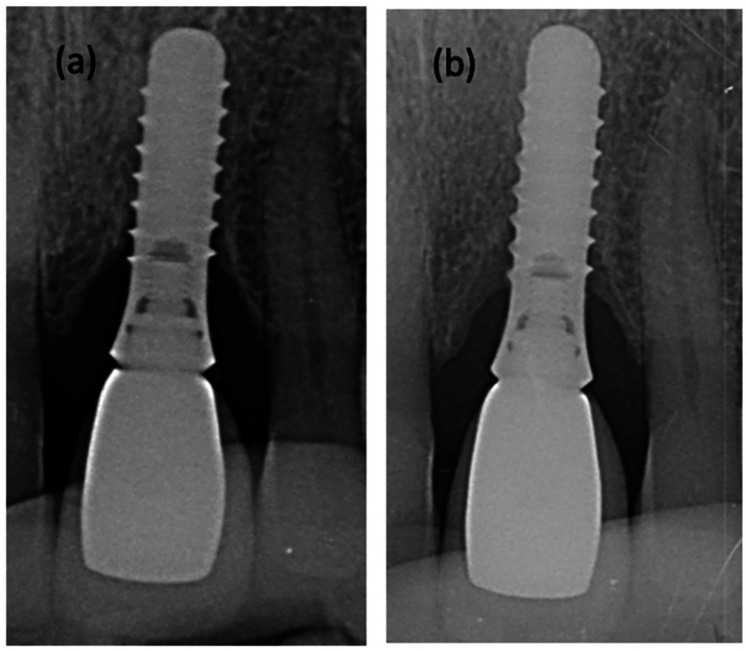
Radiographs of a peri-implant defect (a) before and (b) after non-surgical treatment with glycine air polishing and irrigation of i-PRf.
